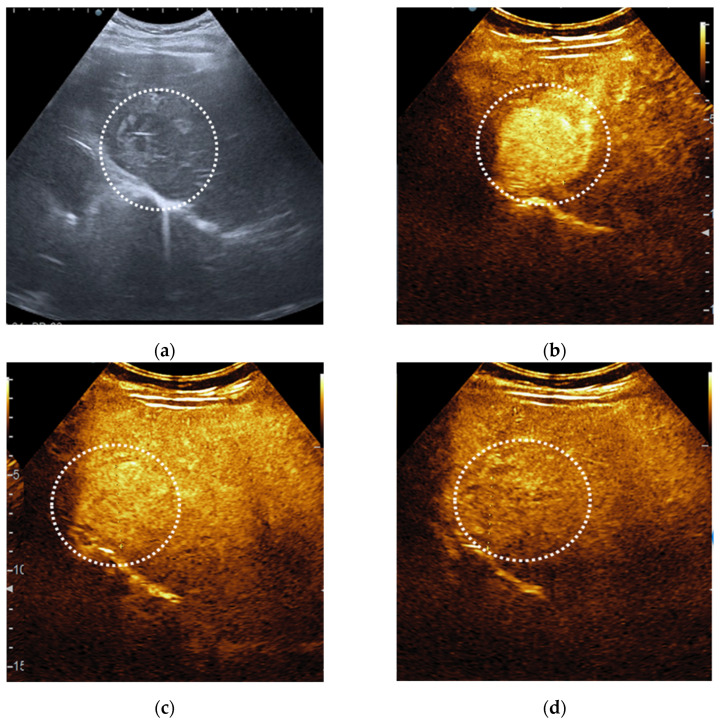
LI-RADS-4 liver lesion, probably HCC. B-mode ultrasound shows an inhomogeneous liver nodule (a). After intravenous administration of contrast agent, an arterial phase hyperenhancement (APHE) is observed (b). No washout is noticed in the portal (c) or in the late phase (d).
